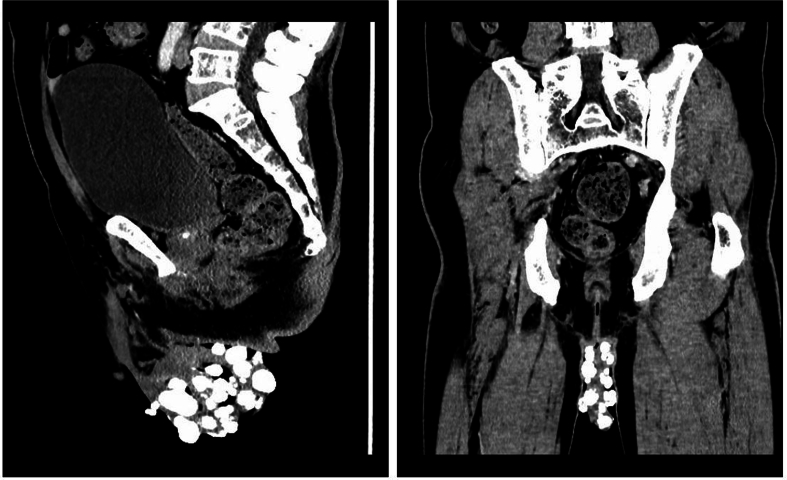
The coronal, sagittal and transversal CT views showed multiple exposed high-density nodules on the skin surface of the scrotum, with clear tubercle boundaries and the largest single tubercle size of 1.5 × 0.9 × 0.7 cm.
